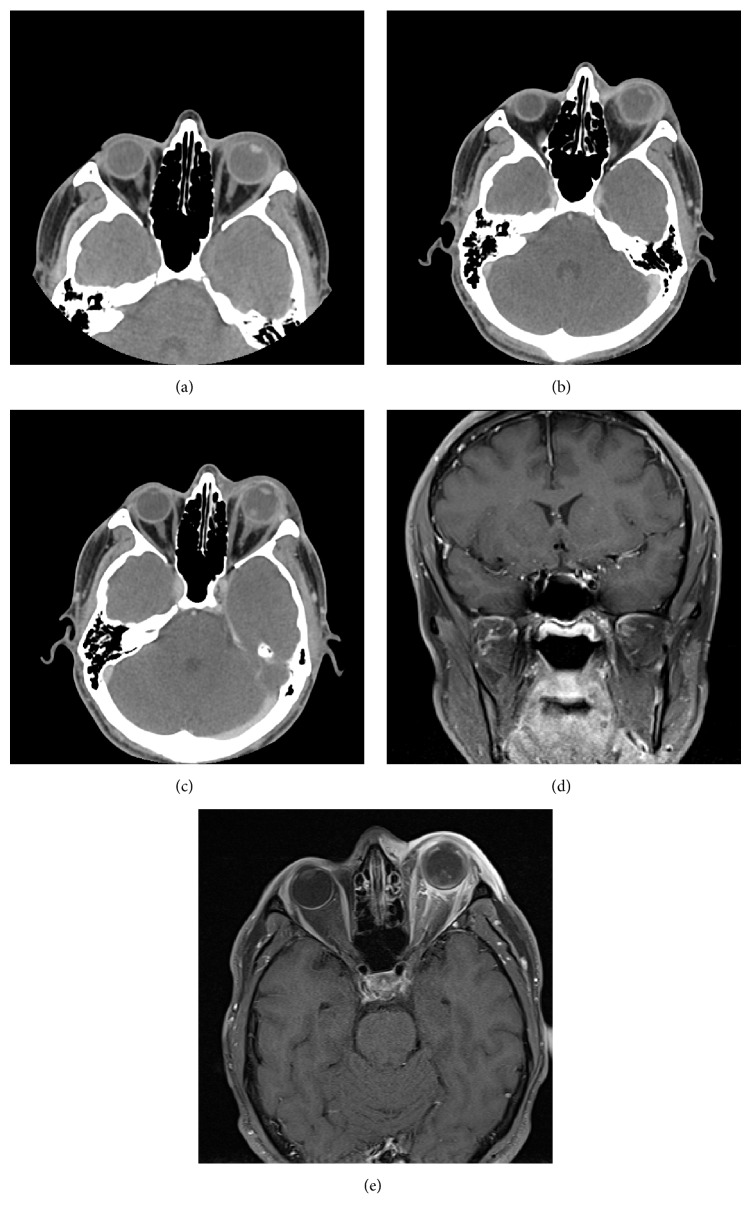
(a) Initial noncontrast CT with left periorbital soft tissue swelling. (b) Follow-up CT-scan with progressive postseptal extension of inflammation with shaggy appearance to the posterior sclera. (c) New vitreous opacity in the posterior globe. (d) MRI with thickening of the globe and scleral enhancement along the inner wall. The native intraocular lens has been displaced into the vitreous. (e) MRI with enhancement along the left optic nerve sheath to the prechiasmatic optic nerve.
